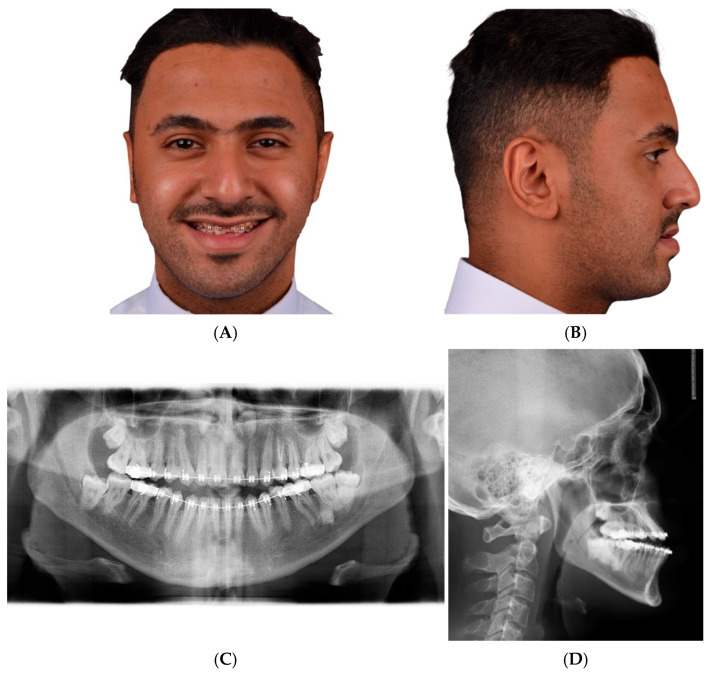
(A) Clinical frontal and (B) lateral photos, (C) panoramic and (D) lateral cephalometric radiographs of the patient. The mandible appears relatively prognathic in the lateral cephalometric radiograph. However, the presence of an impacted third molar is an indication that the jaw is shorter than it should be, which means a retrognathic mandible. More importantly, when compared to the clinical photo, it appears obviously misleading.
